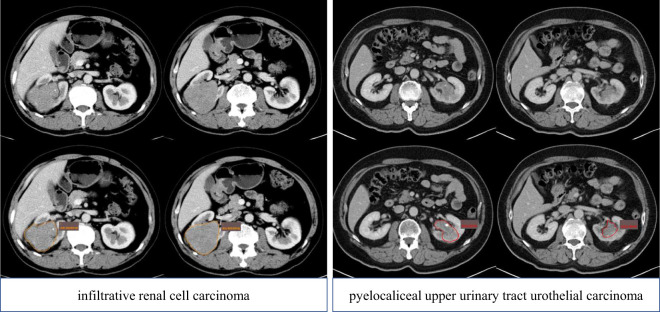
ROI delineation of pyelocaliceal upper urinary tract urothelial carcinoma and infiltrative renal cell carcinoma on the CT (portal phase). ROI, region of interest.
